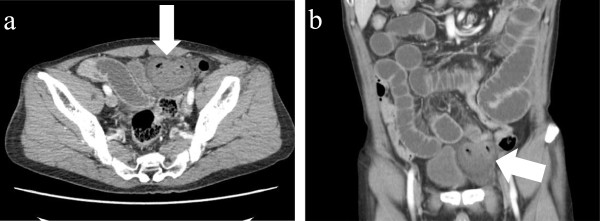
CT findings scan of the patient. Preoperative computed tomography findings of case showing small bowel obstruction with a transition point in the left inguinal groin. A segment of intestine is entrapped in a hernial sac protruding into the pre-peritoneal space; a
